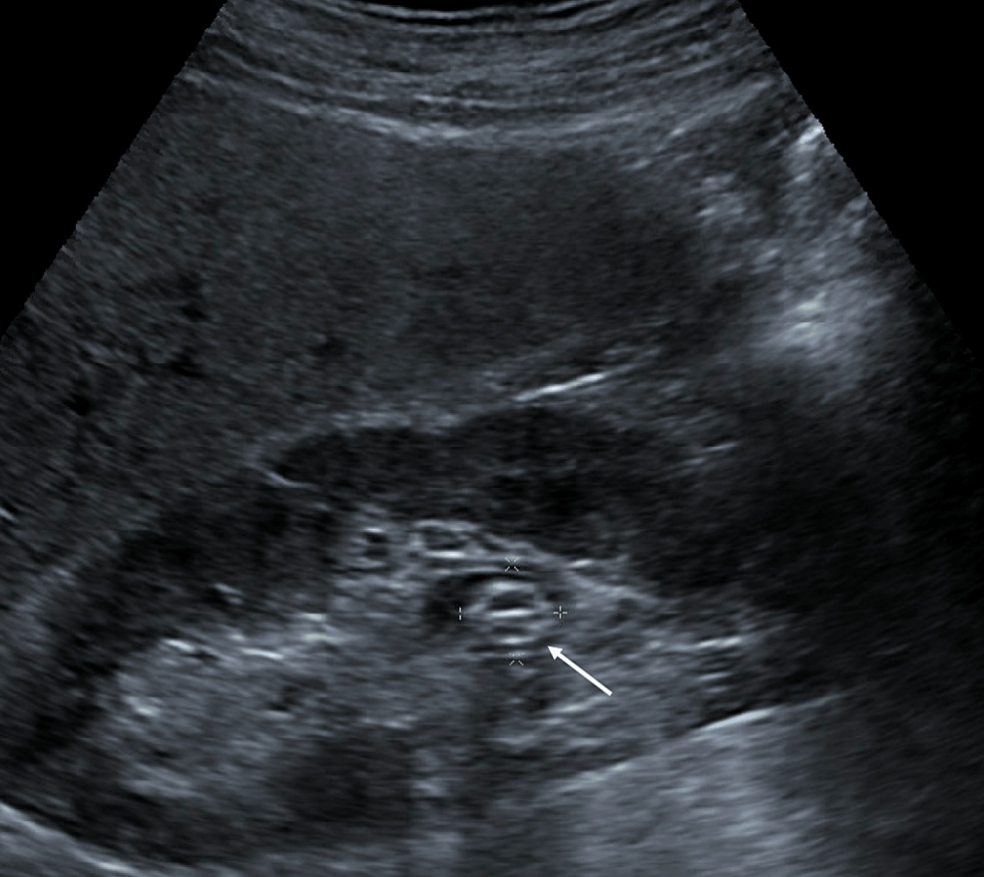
Longitudinal ultrasound image through the right kidney revealed an indeterminate echogenic mass in the right renal pelvis measuring 1.5 cm (white arrow), concerning for recurrent stone, thrombosis, hematoma, or retained encrustation material.
